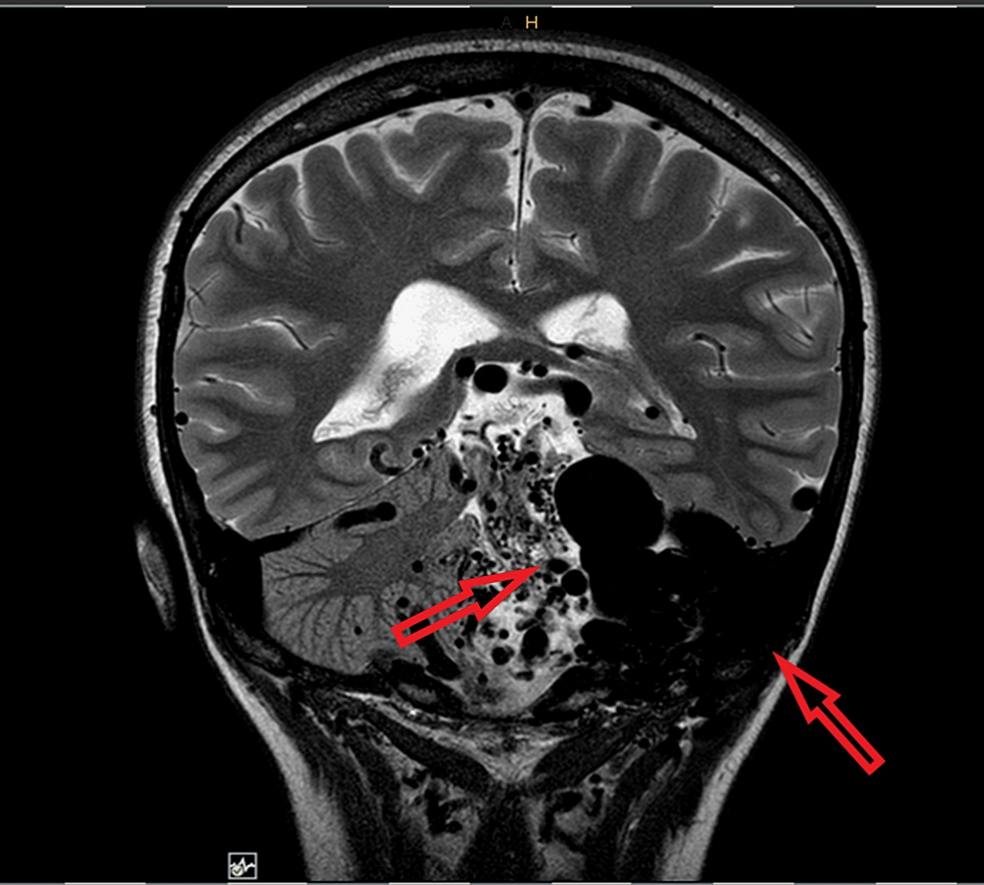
MRI coronal view showing extensive arteriovenous malformation with severe compressing phenomena. The image shows the involvement of posterior fossa structures and left occipital lobe. There is a narrowing of the fourth ventricle and dilation of the ventricular system.
